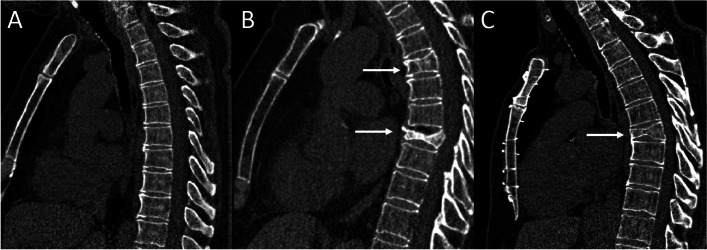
Examples of osteoporotic vertebral fractures in chest CT. A Sagittal view of the thoracic spine with minor degenerations, no vertebral body fractures (B) Osteoporotic vertebral body compression fractures in TH5 (Genant 1) and TH8 (Genant 3) (arrows). C Sintered and beginning wedge-shaped vertebra TH7 (arrow) with compression fracture Genant 2
